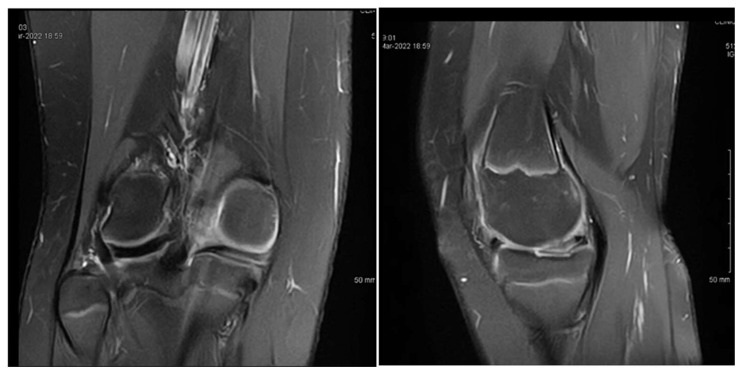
Patient P.E. (12 years old). Follow-up MRI images of the right knee after an initial arthroscopic partial meniscectomy in a pediatric patient with medial discoid meniscus (left—coronal T2 view; right—sagittal T2 view). The coronal image revealed persistent thickening and an abnormal morphology in the medial meniscus, consistent with a residual discoid shape. The sagittal view demonstrated an altered meniscal contour with persistent signal heterogeneity in the posterior horn, suggesting incomplete healing or retearing. Mild joint effusion was still present, indicating ongoing intra-articular inflammation.
